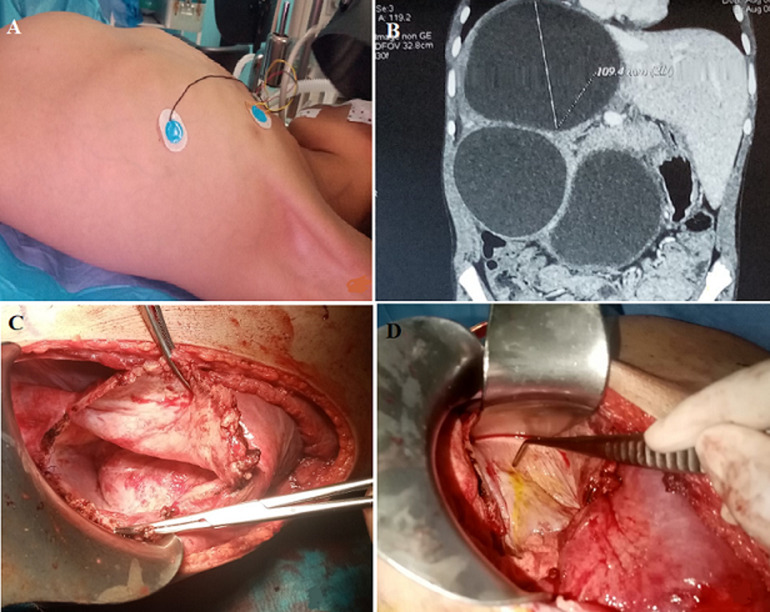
A) abdominal mass; B) abdominal computed tomography (CT) showing multiple huge liver hydatid cysts; C) residual cavity; D) cysto-biliary fistula
